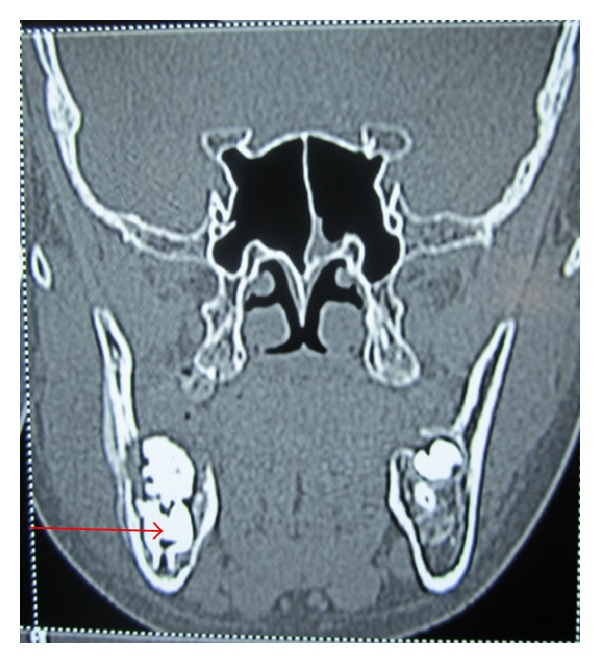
Coronal CT scan section showing the lesion.
